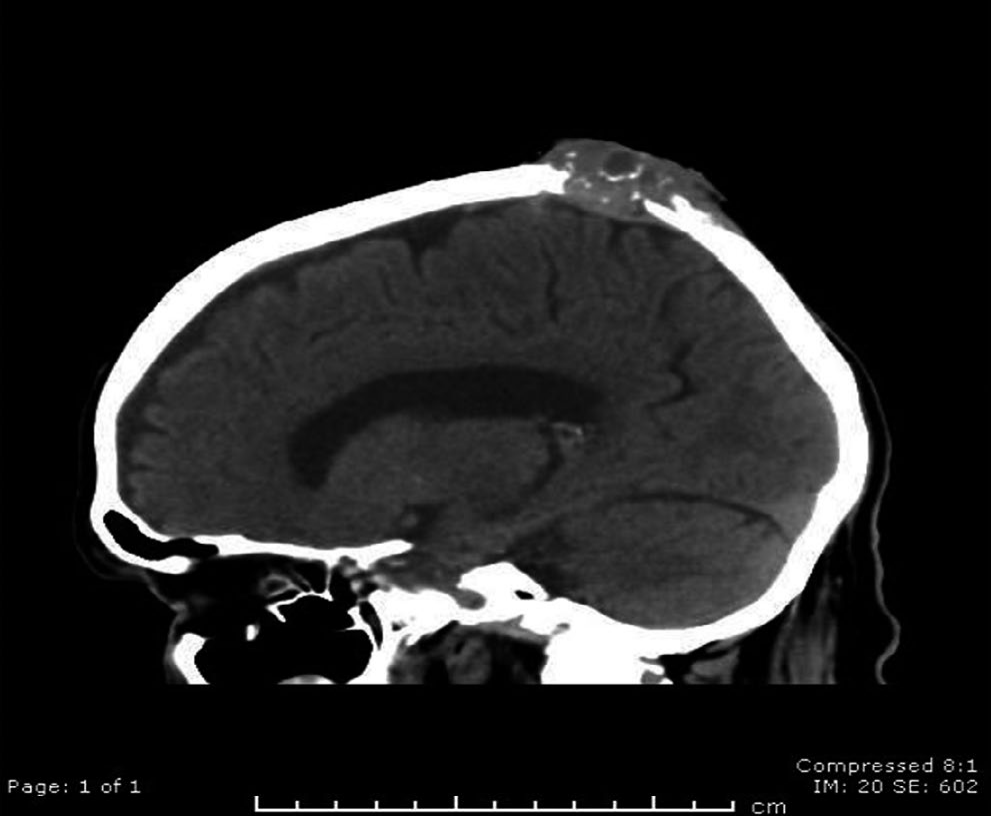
Axial non‐contrast CT image of the skull in bone window showing destructive lesion of the parietal and dura component
